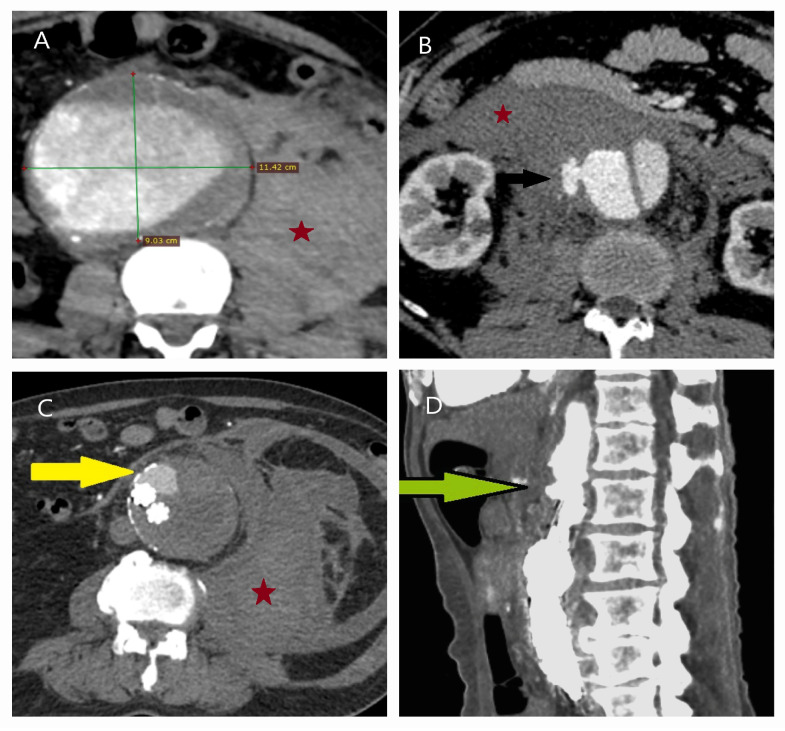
CT images of patients requiring open surgery immediately after EVAR. Three patients—(A) a 72-year-old female, (B) a 38-year-old male with a dissecting ruptured aneurysm (black arrow indicating the rupture site), and (C) a 66-year-old male (yellow arrow indicating a Type 1b endoleak)—developed persistent abdominal distension after EVAR, accompanied by metabolic acidosis. This led to a diagnosis of abdominal tamponade, necessitating open surgery (red stars indicate hematoma). (D) A CT image of a 68-year-old male patient who underwent emergency EVAR for rupture but later required open surgery due to superior mesenteric artery (SMA) thrombosis (green arrow) in the postoperative period. A detailed preoperative evaluation of aortic branches is essential before EVAR, and if necessary, mesenteric anastomoses should be performed via open surgery.
